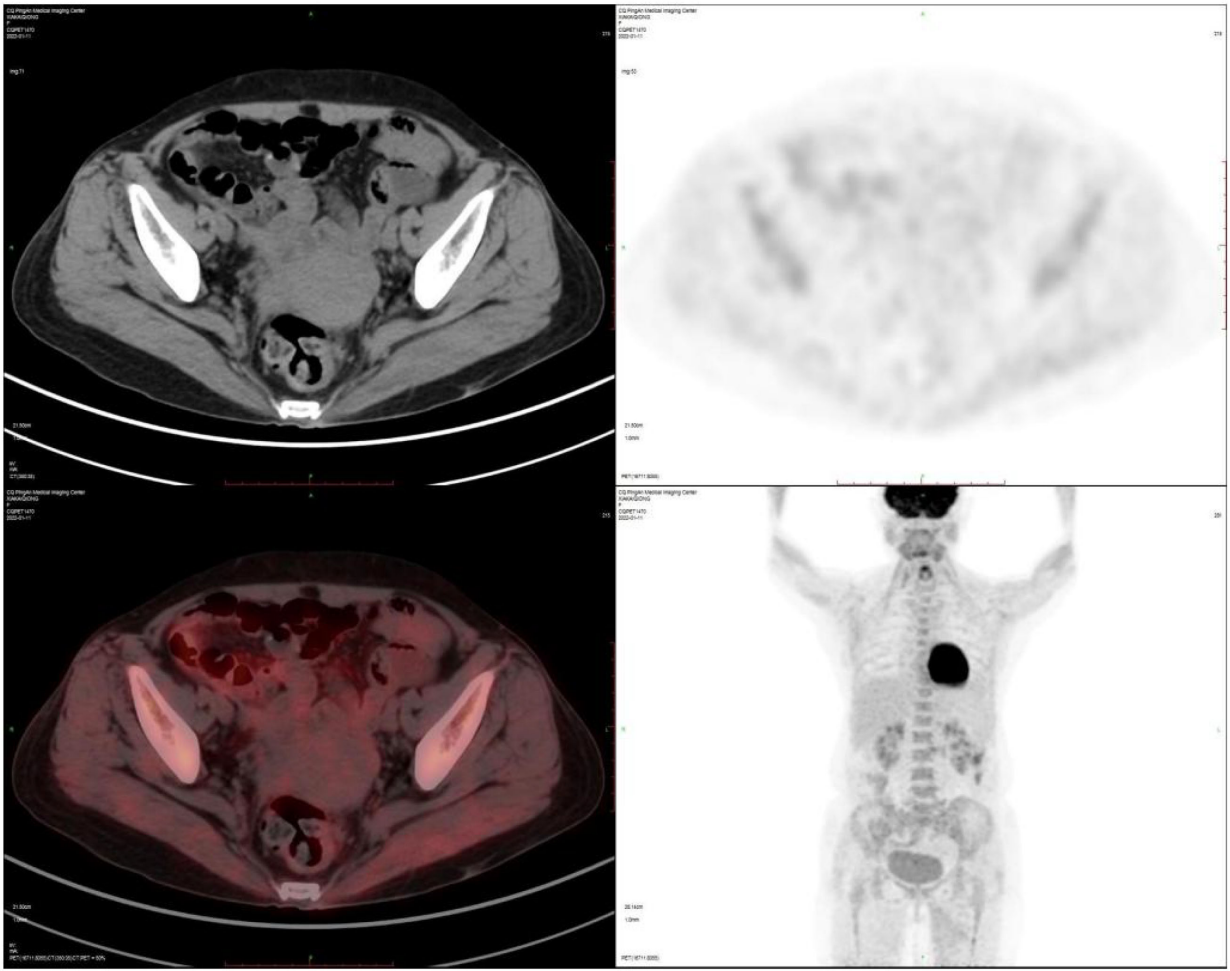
PET-CT at the end of cycle 4 chemotherapy:The lesions in the groin disappeared.
